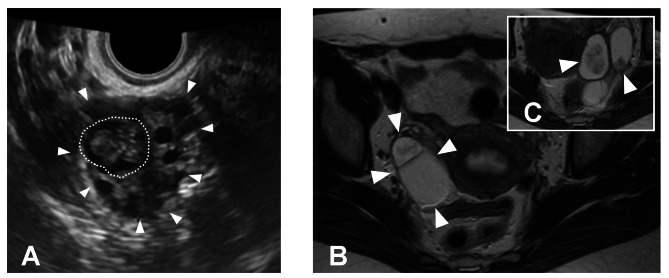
Image findings of the present case. A: Transvaginal ultrasonography. Right ovary (arrowhead) including solid part (dotted line). B, C: MRI (T2-WI). A multifocal mass with a solid area (arrowhead) was observed in the right ovary (B), closely resembling that on preoperative MRI for a left ovarian tumor (C, arrowhead)
